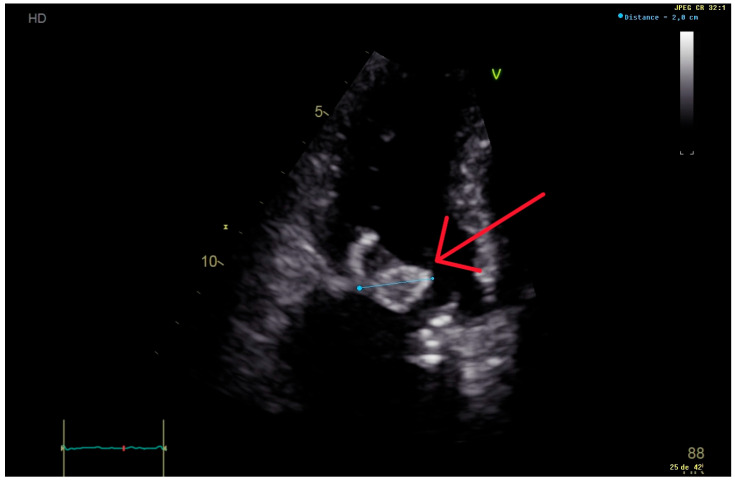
Trans-thoracic echocardiography performed 6 months after the first valvular replacement, showing a 20 mm vegetation on the mitral prosthetic valve (red arrow), causing severe intraprosthetic obstruction.
